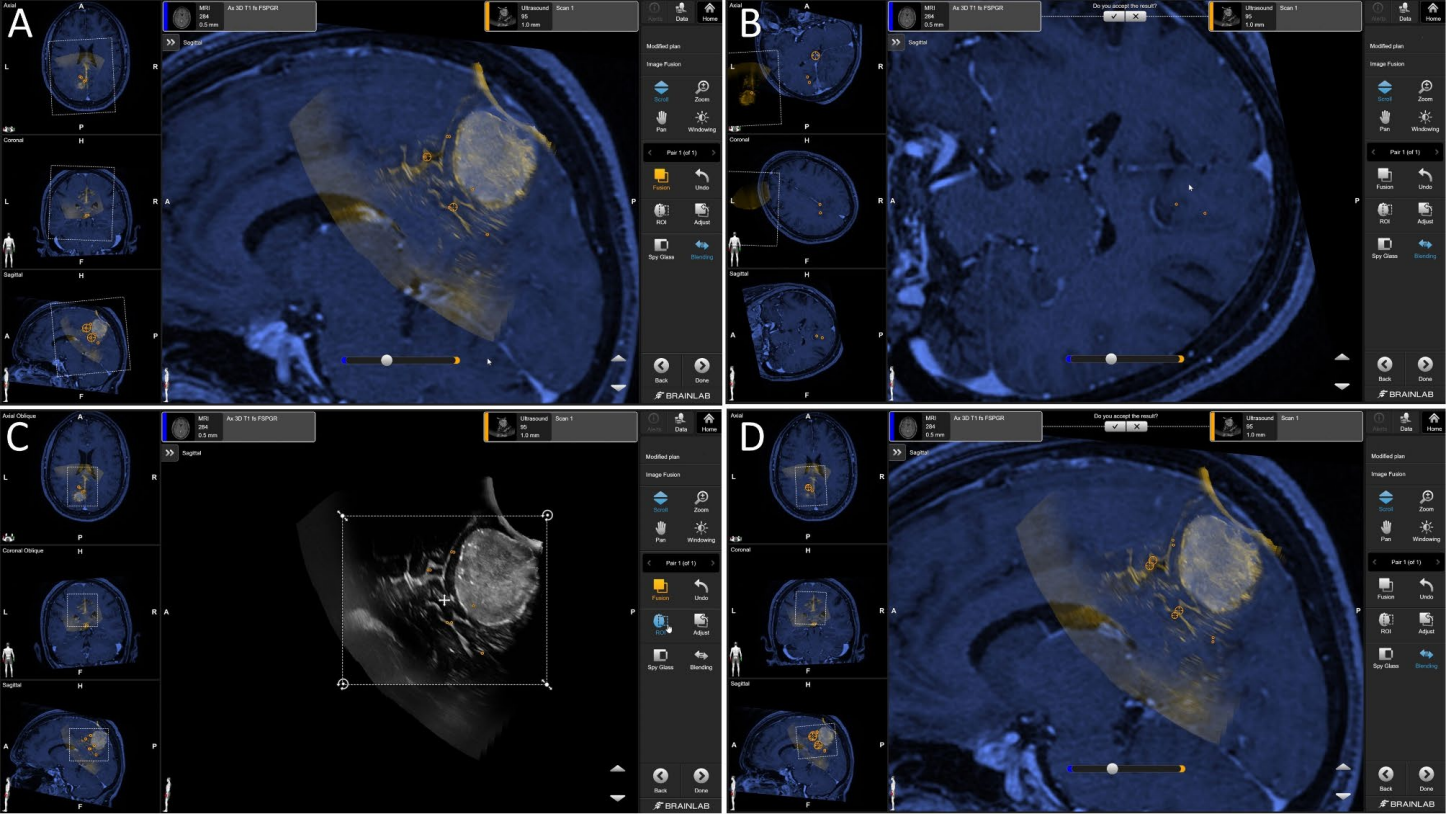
Patient dataset excluded from quantitative evaluation of the automatic image fusion algorithm. This dataset showed initial misalignment of the RBF (A) and the algorithm using a default fusion region of interest (ROI), which is determined based on the field of view of the ultrasound scan was not able to align both datasets in fully automated manner (B). After manual definition of the fusion ROI – focusing on the clinical target and some deep anatomical structures serving as references for the RIF (C) – the proposed method was able to provide a highly accurate co-registration result (D)
